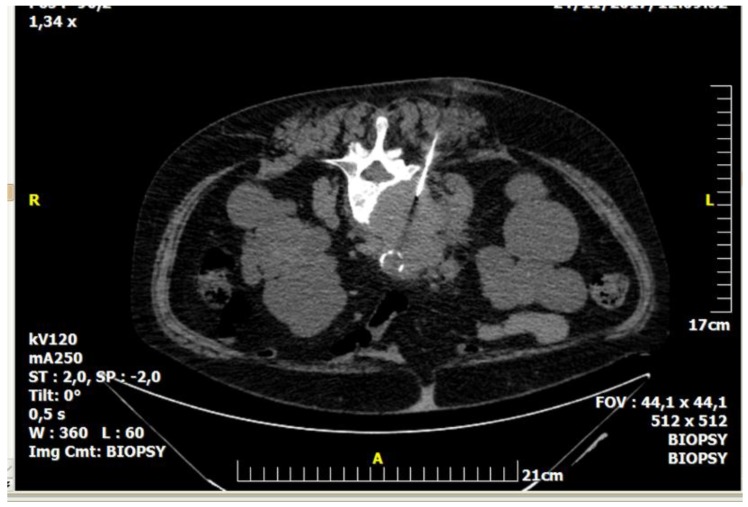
A 59-year-old female patient with a medical record of urothelial carcinoma. Computed tomography axial scan: there is a soft tissue mass infiltrating the L4 vertebral body. The result of percutaneous, imaging-guided biopsy was metastasis from urothelial carcinoma.
